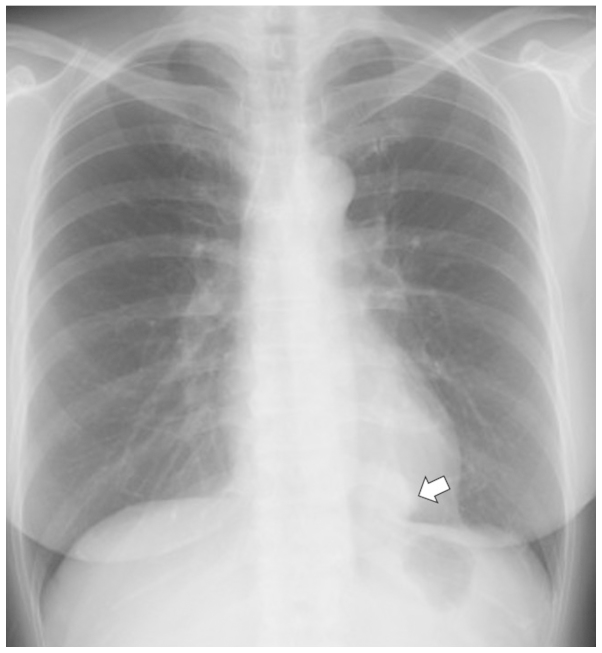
Mass shadow (arrow) detected on a chest radiograph during a mass-screening program.
